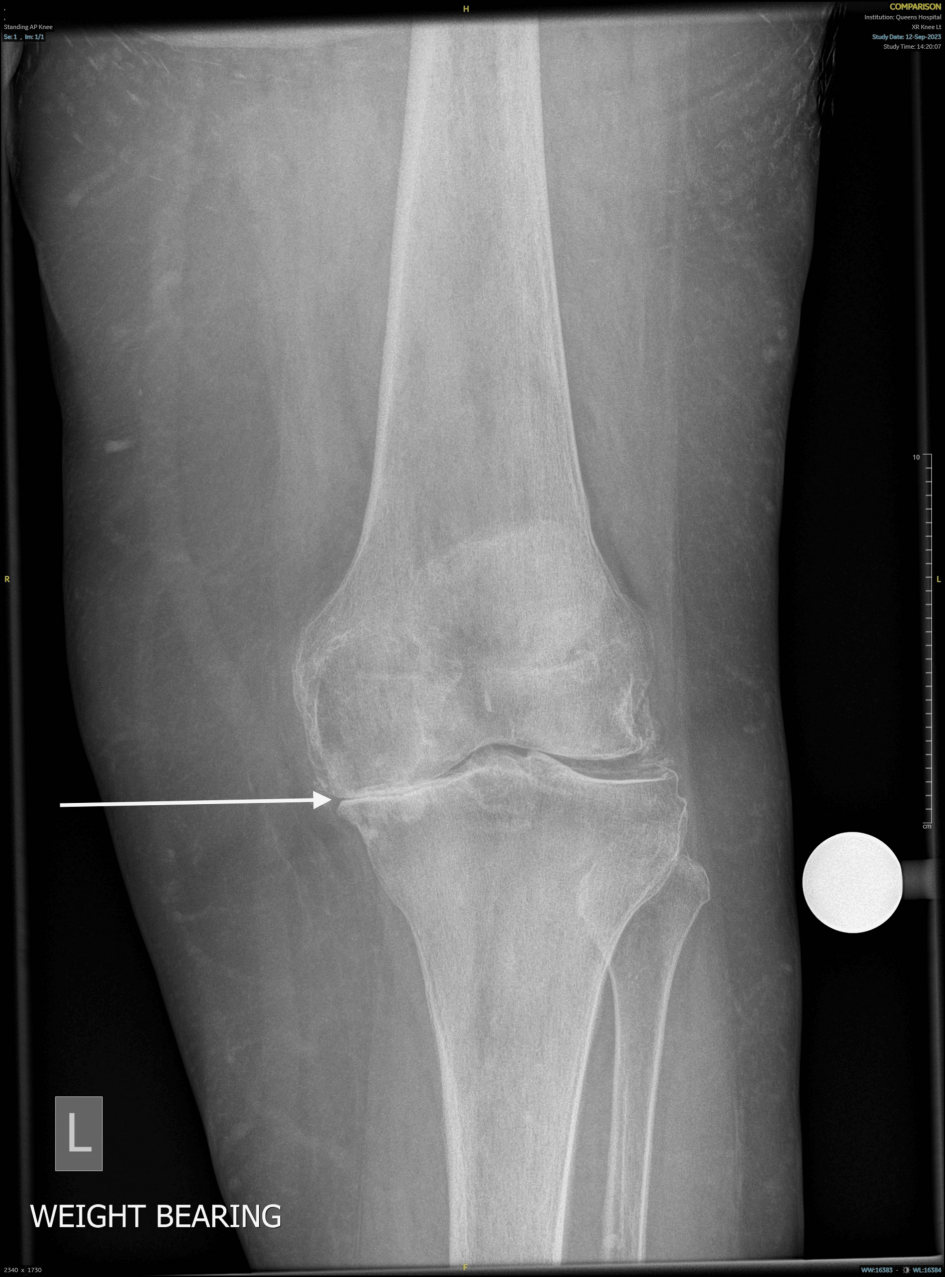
Preoperative anteroposterior radiograph of the left knee showing degenerative changes of the joint prior to total knee replacement The arrow indicates degenerative changes of the knee joint consistent with osteoarthritis.
